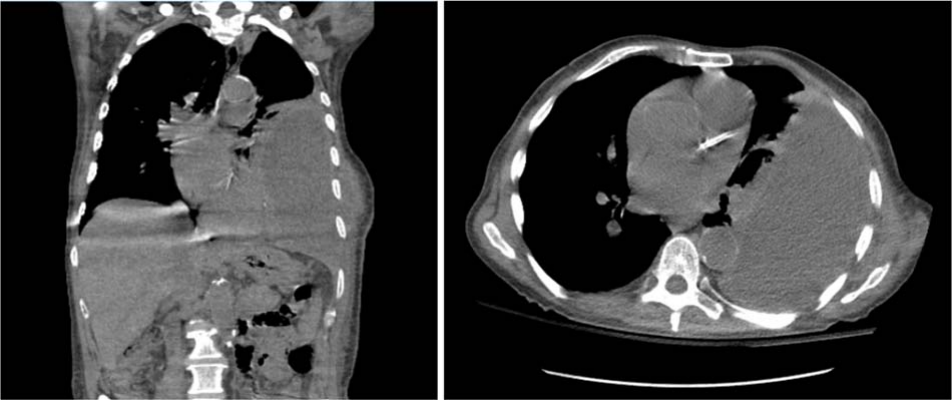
Coronal (left) and axial (right) view CT scan of the chest showing a large complex loculated left pleural effusion with bulging into the chest wall, raising concern for empyema necessitans.
